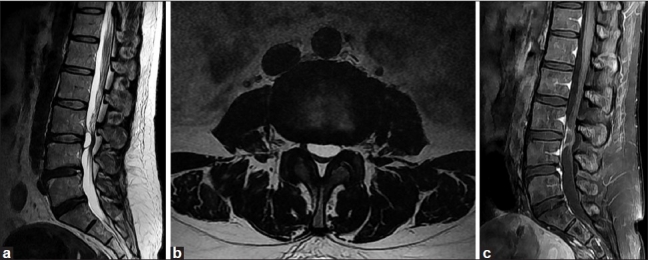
Sagittal (a) and axial (b) T2-weighted MRI demonstrating a cystic lesion at the level of L3-4 intervertebral disc. In sagittal (c) T1-weighted MRI, rim enhancement of the lesion was appreciated after gadolinium administration
